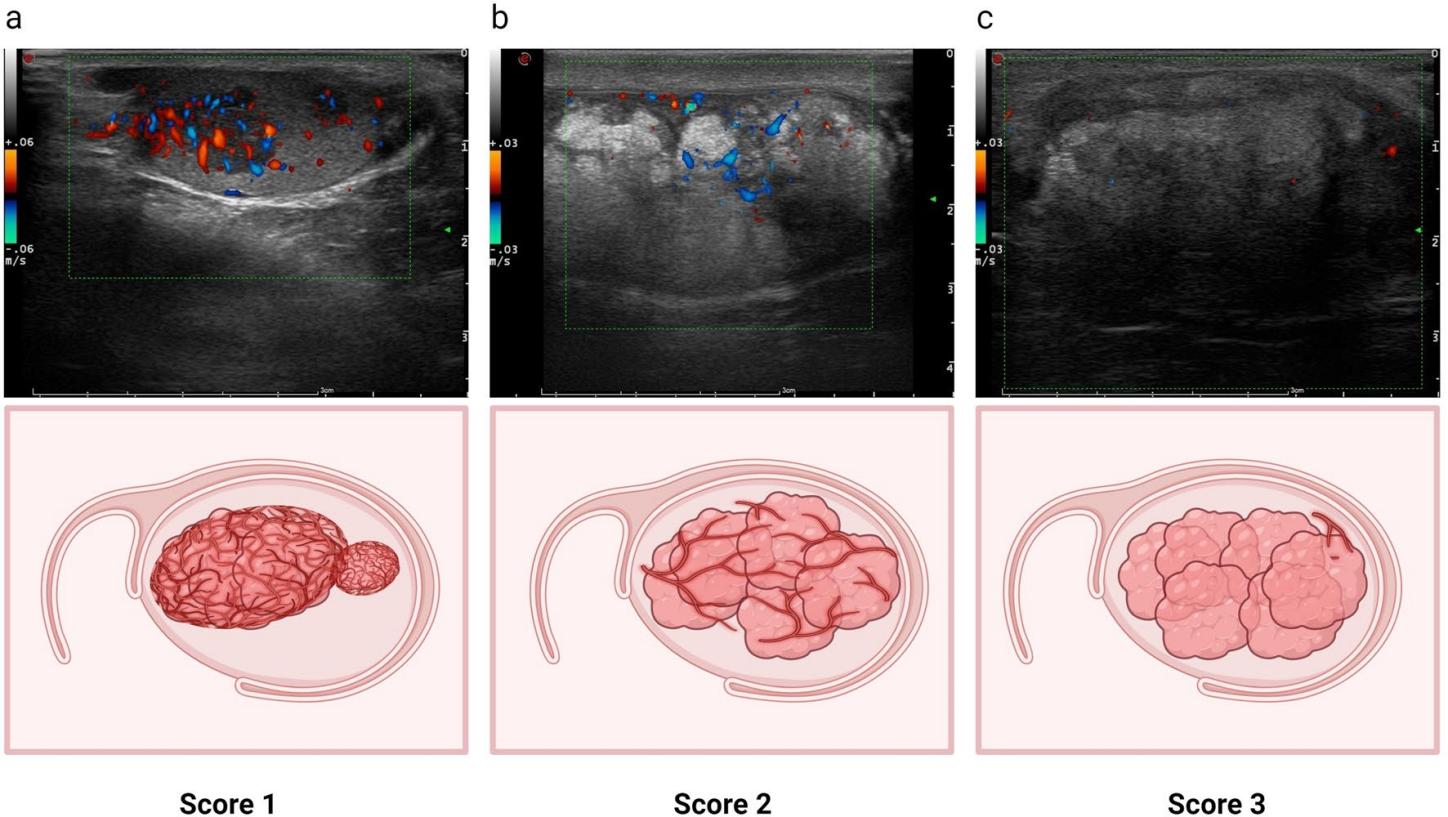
Typical images of testicular adrenal rest tumor (TART) vascularity patterns scores. (a–c) depict testicular power Doppler images of male CAH patients aged 13, 41, and 38, respectively. (a) Score1: Abundant linear blood flow signals filling the entire lesion; (b) Score 2: Moderate linear blood flow signals occupying part of the lesion; (c) Score 3: No blood flow or very limited blood flow around lesion.
